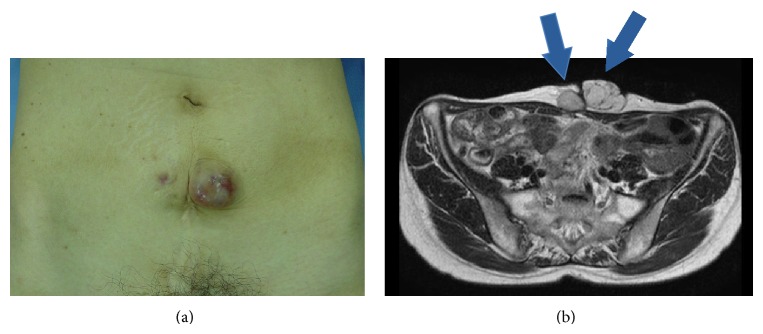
(a) Smooth mass on the middle-left side of the cesarean median scar. (b) Pelvic MRI (T2 weighted image, axial section). MRI shows the tumor associated with cesarean section scar. The right side of the scar consists of solid components and the tumor at the left side of the scar is polycystic.
